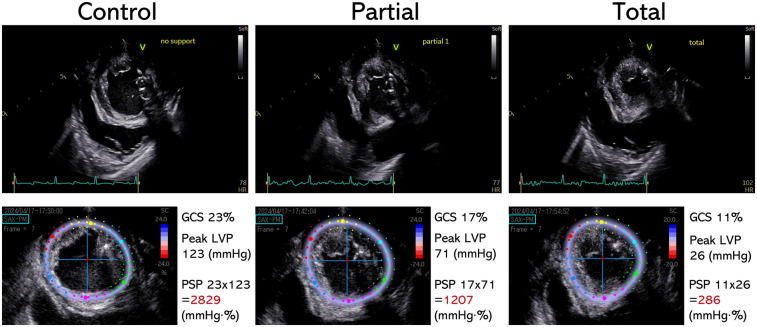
Representative echocardiographic images and PSP calculation. Upper pictures: Echocardiographic images of left ventricular short axis view at the mid-papillary level. Lower pictures: Speckle-tracking echocardiographic analysis corresponding to the upper pictures. PSP at each LVAD support level is shown as the product of GCS and peak LV pressure. GCS, global circumferential strain; LVAD, left ventricular assist device; LVP, left ventricular pressure; PSP, pressure-strain product.
